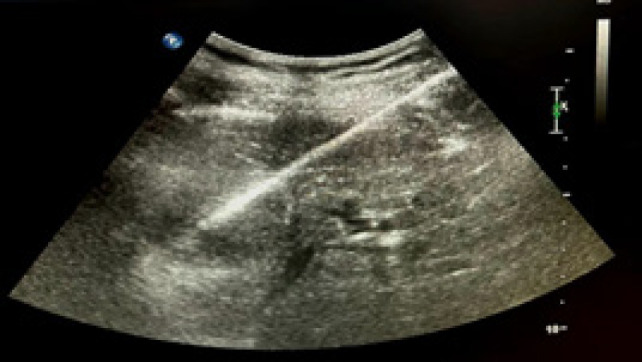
Ultrasound image of renal biopsy before ablation.
